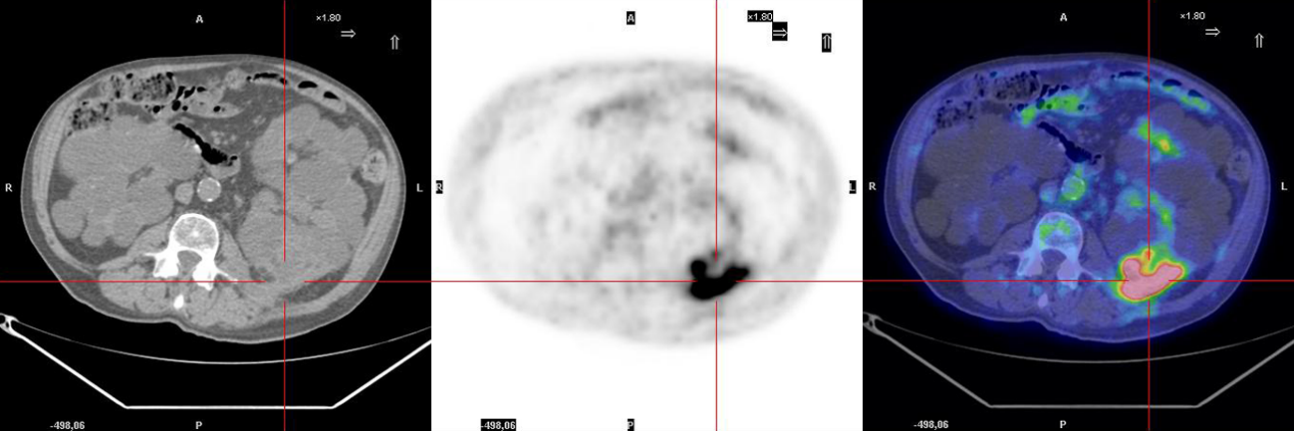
FDG-PET scan: intracystic kidney infection in ADPKD.
